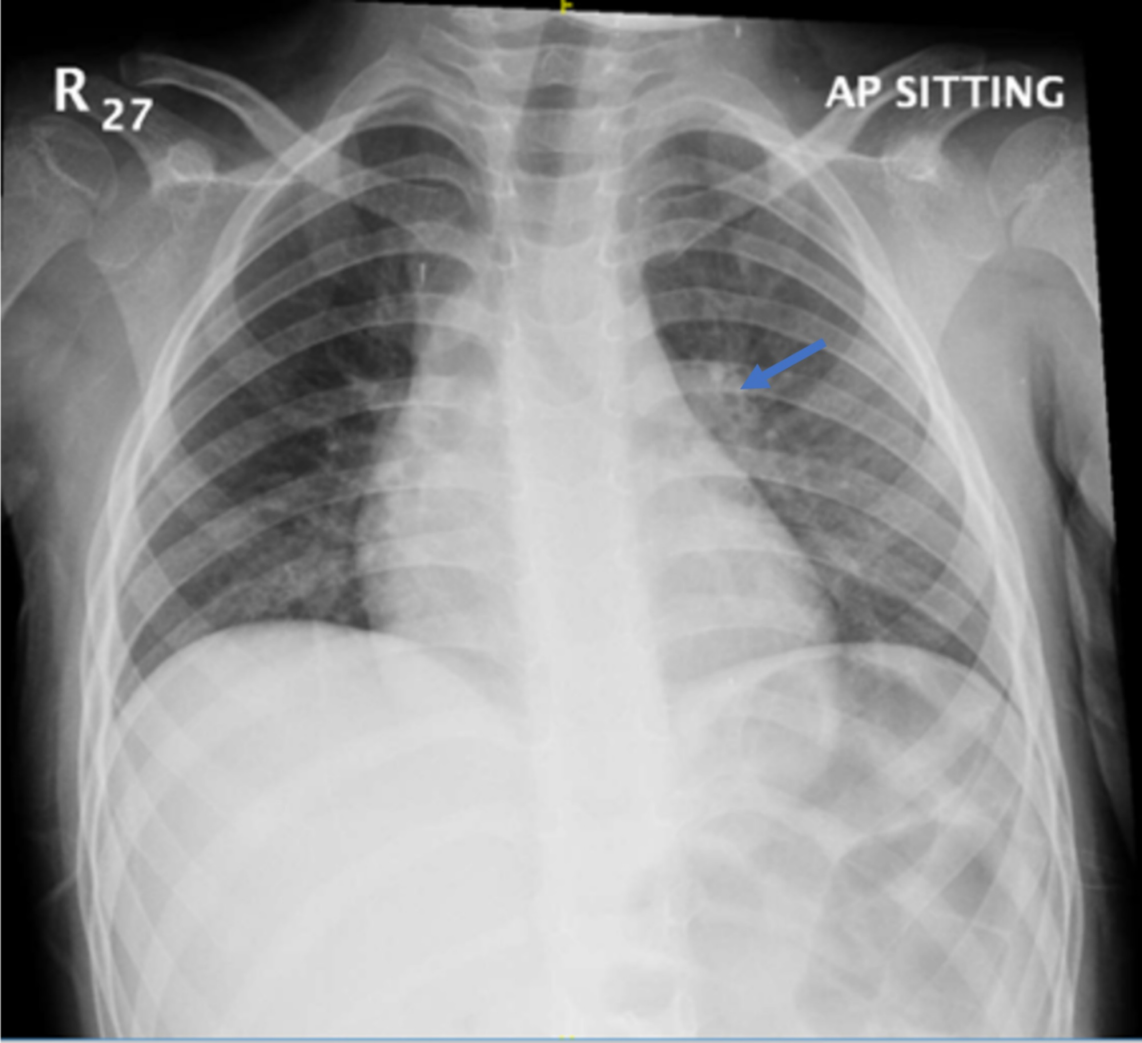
Chest X-ray of the patient The arrow demonstrates peri-bronchial wall thickening indicating small airway disease
